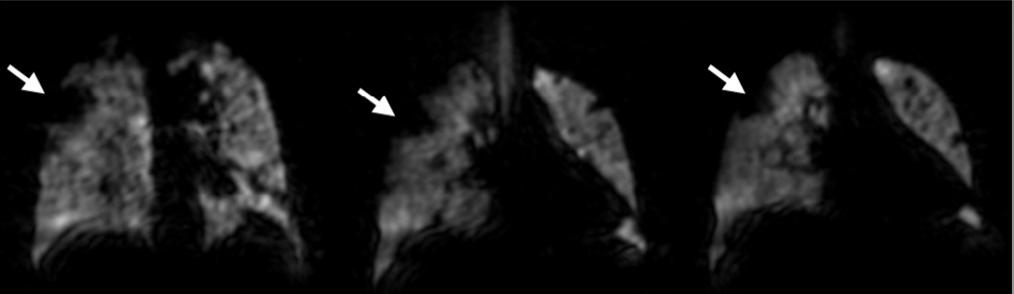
Patient I: Undifferentiated airways disease. A 71-year-old ex-smoker with progressive breathlessness on minor exertion and hypothyroidism. Spirometry was supranormal with no acute reversibility to salbutamol. No gas trapping or hyperexpansion. Normal echocardiogram. CT showed minor emphysema, disproportionate to the extent of abnormality in gas transfer (TL_CO_ 47.9%). No acute or chronic clot on CT pulmonary angiography. ^1^H perfusion MRI showed minor abnormalities. HP gas ventilation MRI showed multiple predominantly segmental ventilation defects most prominent in the right upper lobe (white arrows). MRI determined that there were substantial ventilation abnormalities despite normal spirometry, alongside relatively normal perfusion, and therefore poor local V/Q matching.
